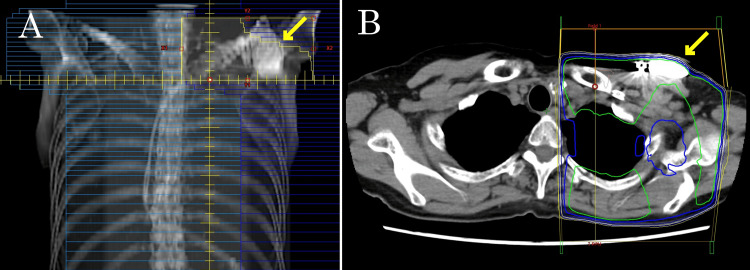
The irradiation field for the left supraclavicular region directly overlaps the pacemaker implanted subcutaneously in the left anterior chest wall. (A) Digitally reconstructed radiograph of the supraclavicular field showing overlap with the pacemaker. (B) CT image with dose distribution showing direct radiation exposure to the pacemaker.
